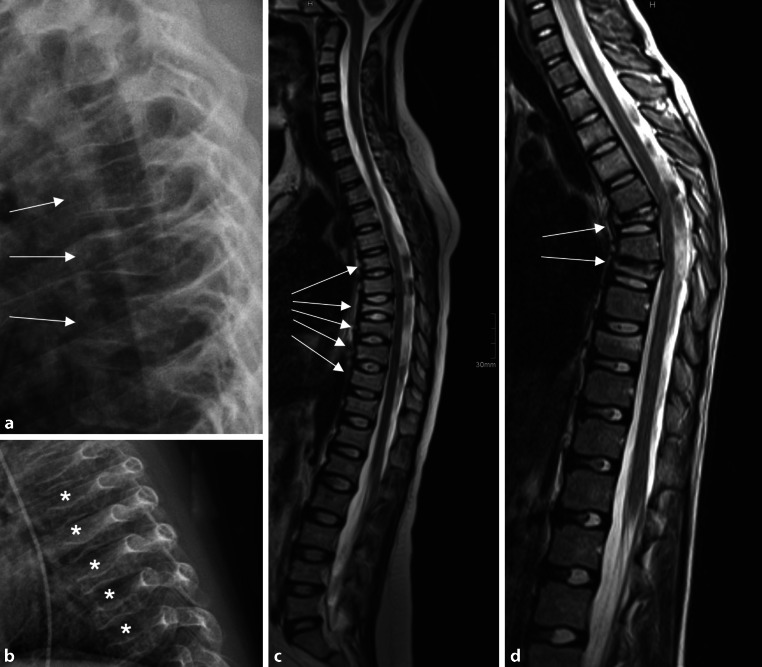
Pediatric and adolescent spine fractures. a Osteogenesis imperfecta. *Arrows *fractured vertebrae (anterior wedging). b Secondary osteoporosis (after heart transplantation). *Asterisks* indicate the preserved and relatively wide disc spaces, a typical feature of pediatric spinal insufficiency fractures. c Idiopathic osteoporosis. Vertebral fractures (*arrows*) on magnetic resonance imaging (MRI). d Chronic non-infectious osteomyelitis (CNO)/chronic recurrent multifocal osteomyelitis (CRMO). Severe fractures (*arrows*) leading to thoracic gibbus deformity
